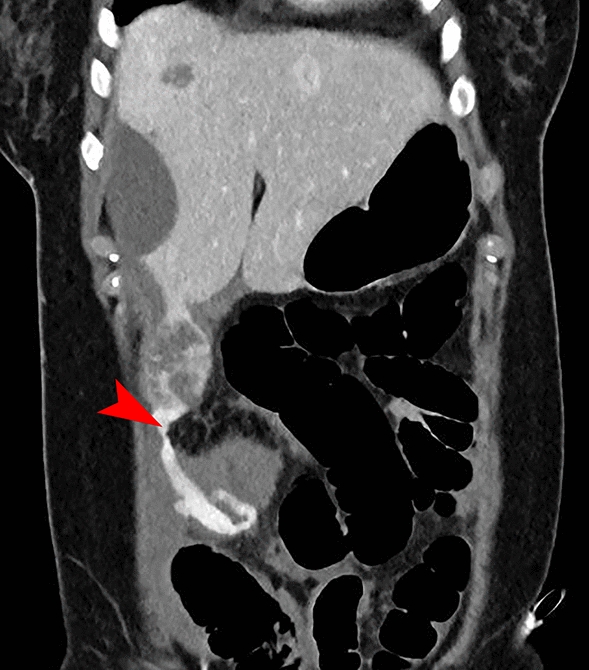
This abdominal computed tomography revealed a new active bleed from the adenoma in segment 6, with a newly developed extravasation (marked with red arrow) into the free abdominal cavity: the right paracolic region behind the right rectus abdominis muscle
